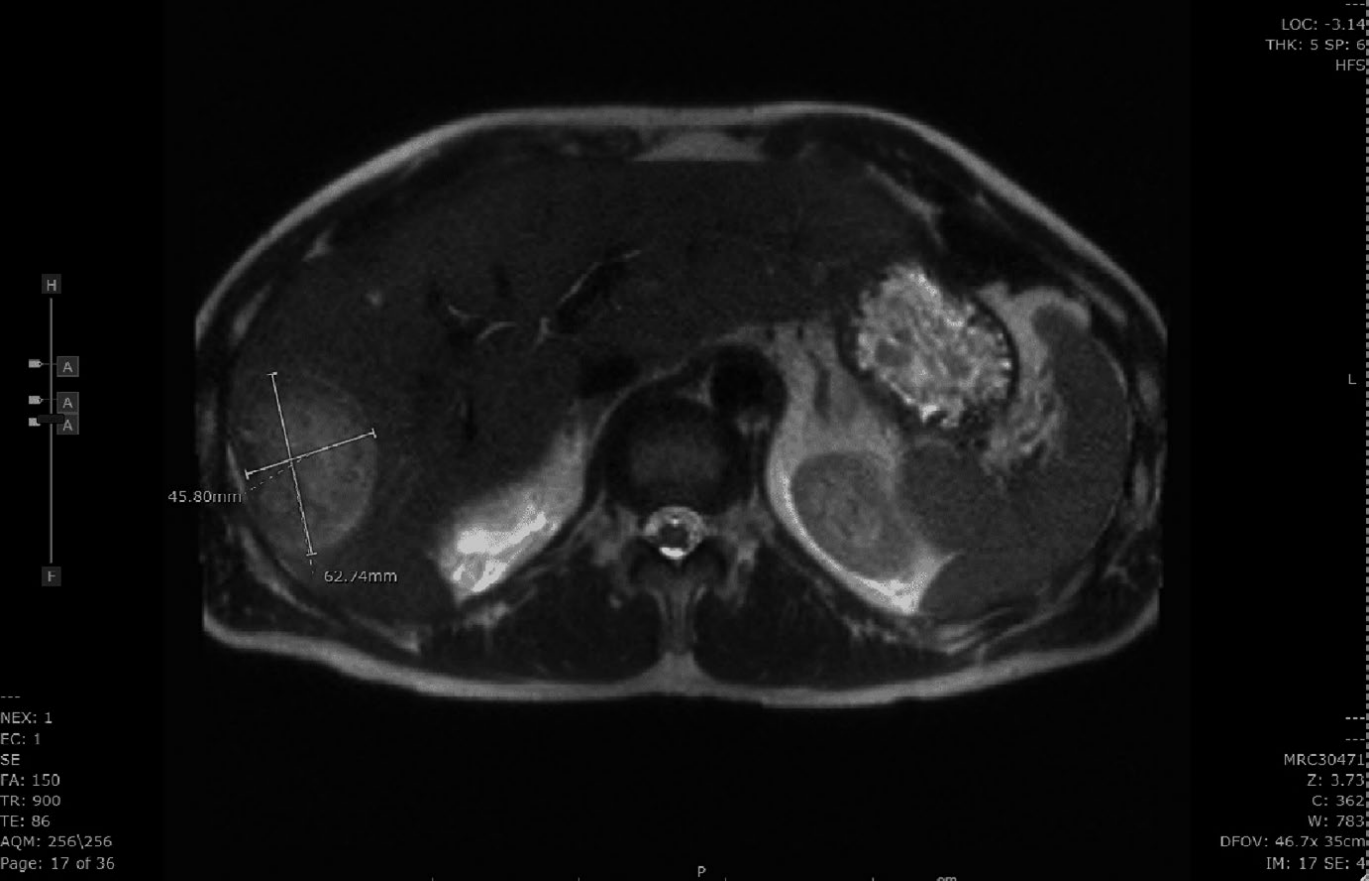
Magnetic resonance imaging (MRI) scan, during hospitalization, showing heterogeneous mildly T2 hyperintense and T2 hypointense lesion within the right hepatic lobe measuring 4.6 × 6.3 × 6.0 cm (transverse by AP by craniocaudal length), with a continuous thick irregular wall and internal solid and cystic components, with vascular enhancement of the wall and internal solid components
